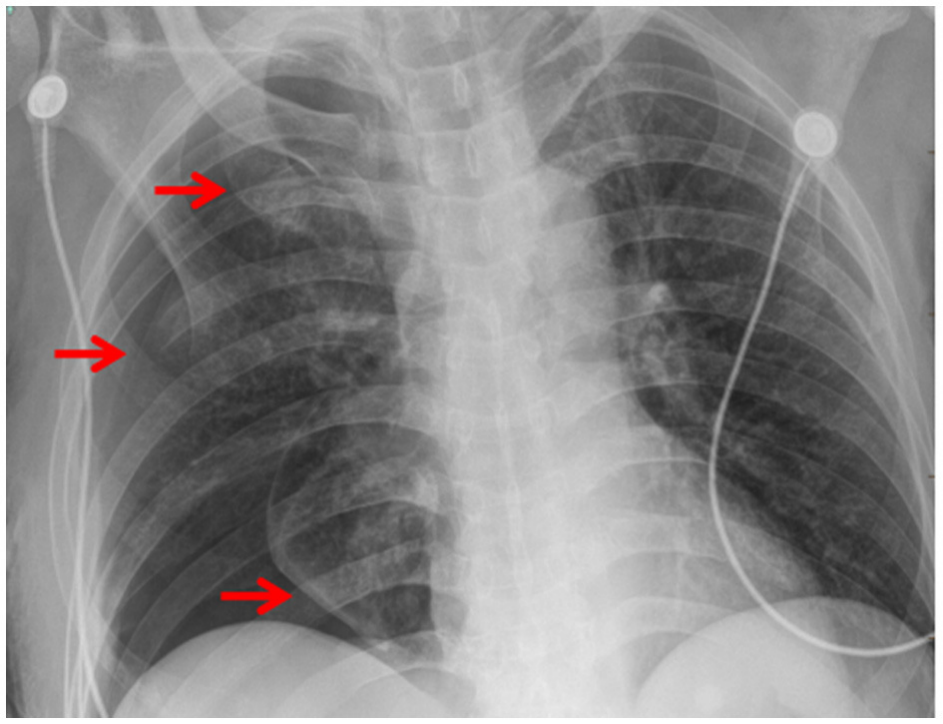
Pneumothorax was presented after the removal of the chest tube in x-ray (red arrow).
